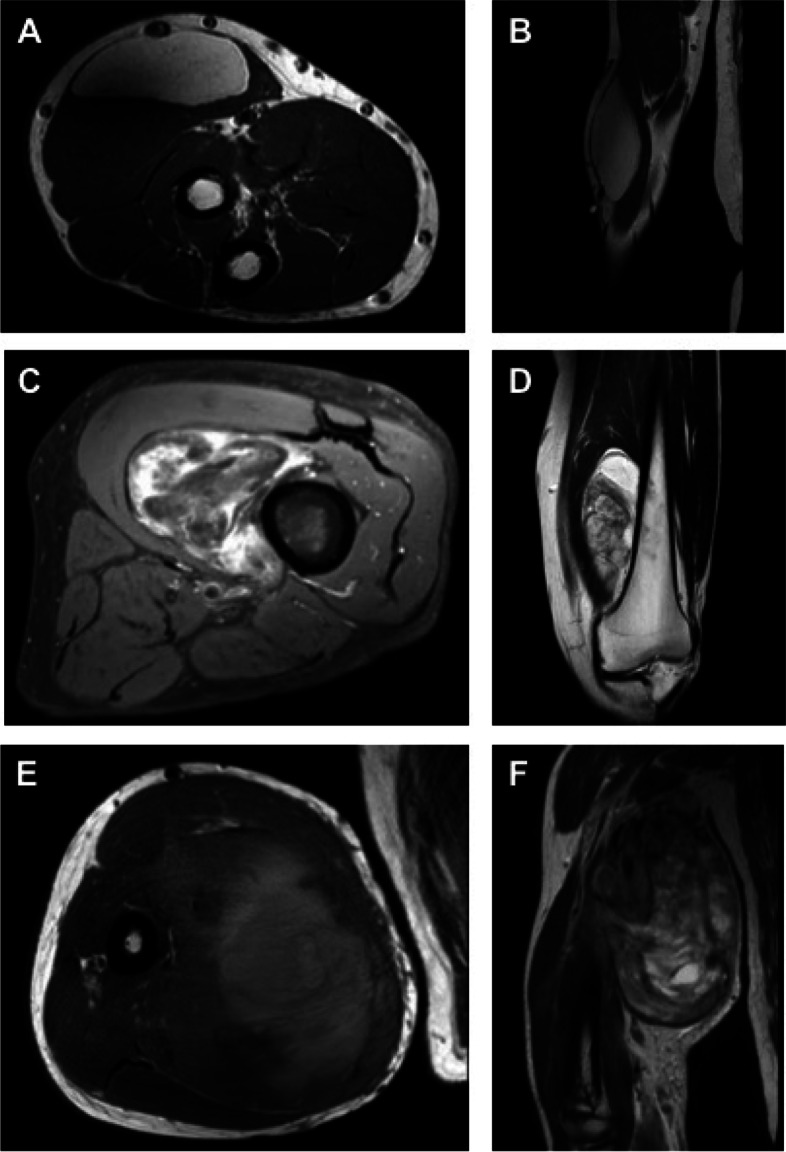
Examples for lipomatous tumors in MRI. A Lipoma in a T1-weighted sequence in transversal plane. B Corresponding T2-weighted coronal image. C Atypical lipomatous tumor (ALT) in a contrast-enhanced fat-saturated T1-weighed sequence in transversal plane demonstrating inhomogeneous contrast enhancement. D Corresponding T2-weighted coronal image. E Pleomorphic liposarcoma in a T1-weighted-sequence in axial plane. F Corresponding T2-weighted coronal image
